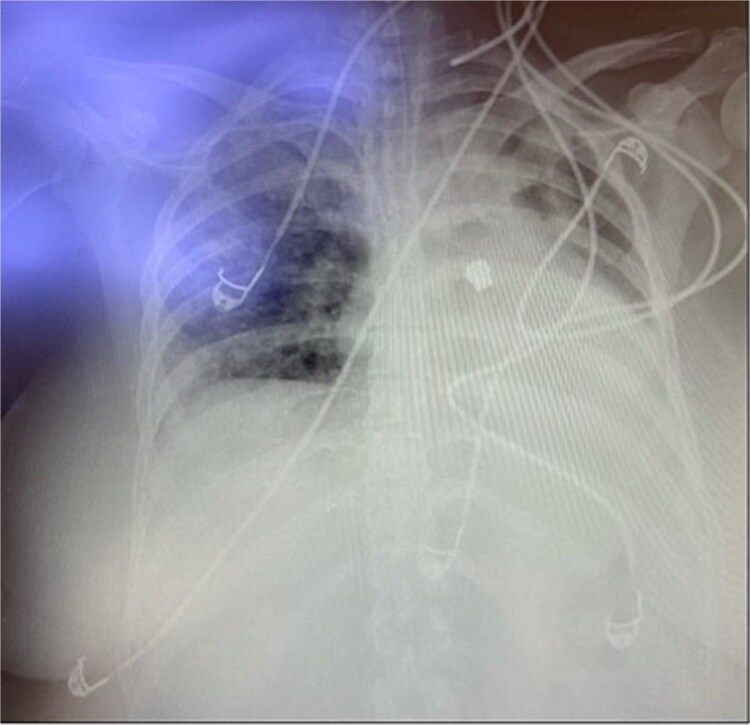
X-ray of the chest showing the foreign body in the left chest.
